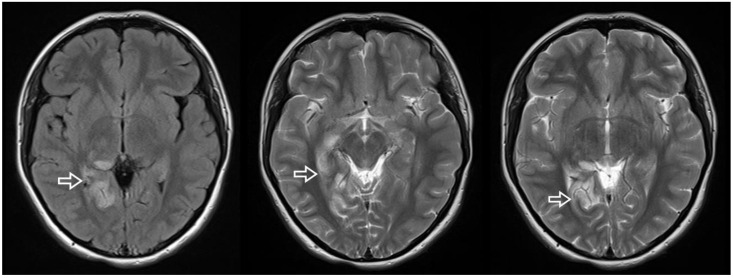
Brain MRI axial sections showing the ischemic lesions in the territory of the right posterior cerebral artery (arrow).
